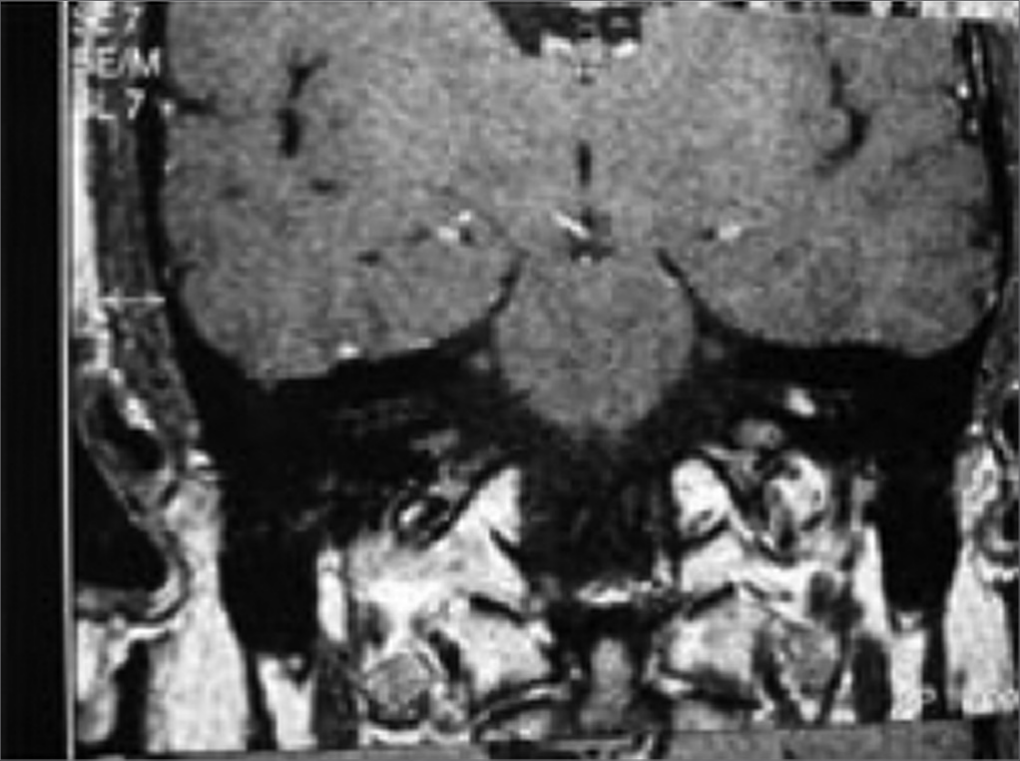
MRI with Vestibular Schwannoma, coronal view.
